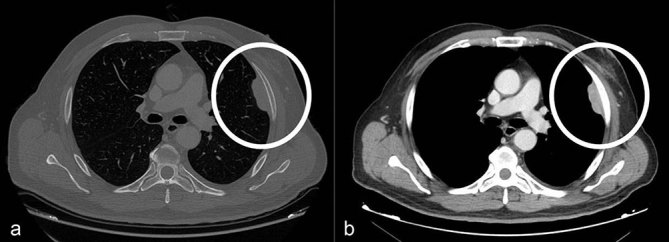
(a, b) CT scan of the thorax after i.v. contrast showing a solitary well-circumscribed, homogeneous solid subpleural mass (circles) in the upper left hemithorax with an obtuse angle between the mass and the chest wall. No rib invasion was noted.
